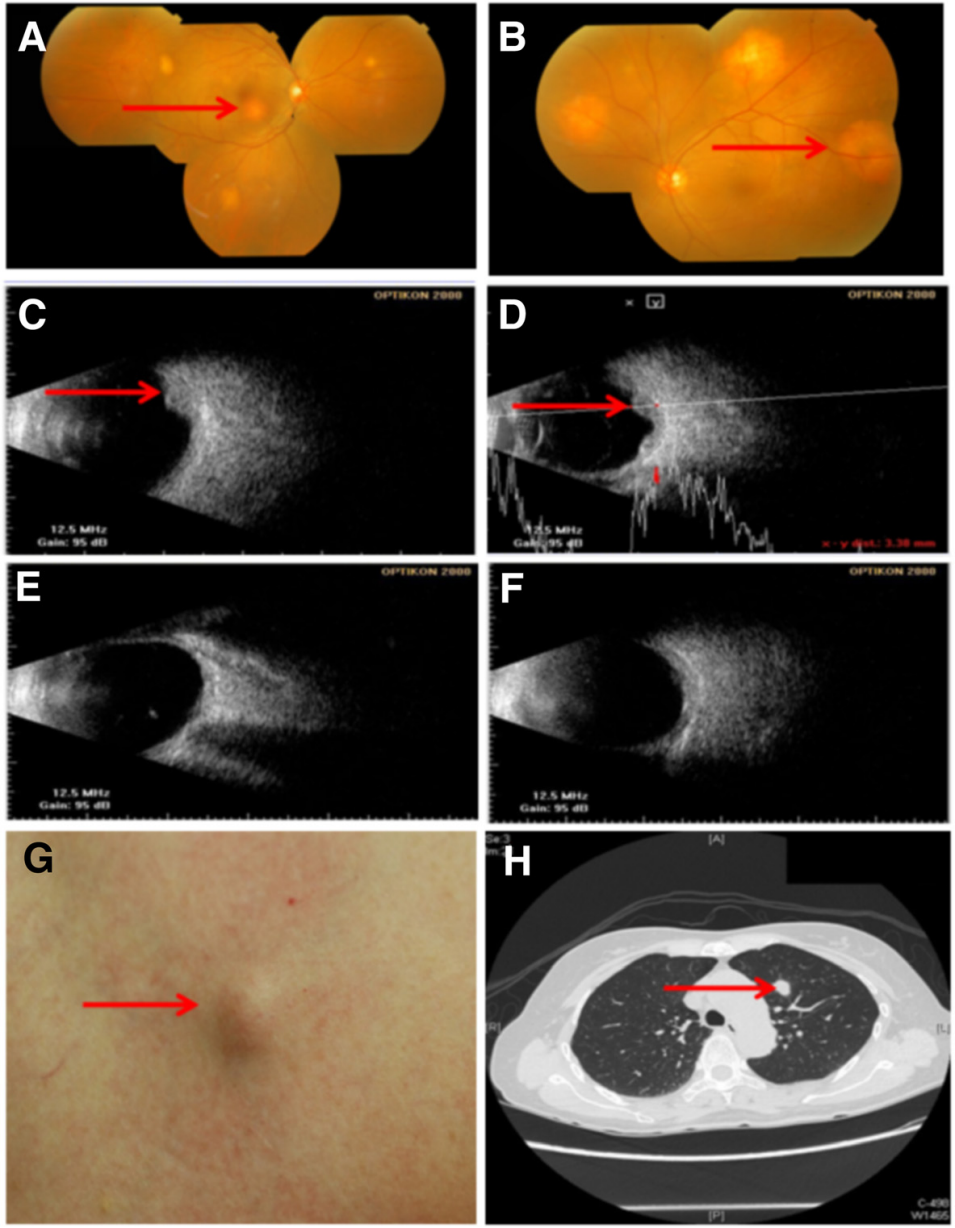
Choroid and skin metastases of primary clear cell adenocarcinoma of lung. (A-F) Ophthalmology images; (A,C,E) right eye, (B,D,F) left eye. (A,B) Fundus appearance before treatment (arrows point to lesions); (C,D) ultrasound scan of the same eyes as in (A,B) before treatment (arrows point to lesions); (E,F) lesion resolution by ultrasound scan of the same eyes as in (A,B) after treatment. (G) Appearance of skin metastasis (arrow). (H) Chest computed tomography scan showing left upper lobe mass (1.3cm in diameter) (arrow).
